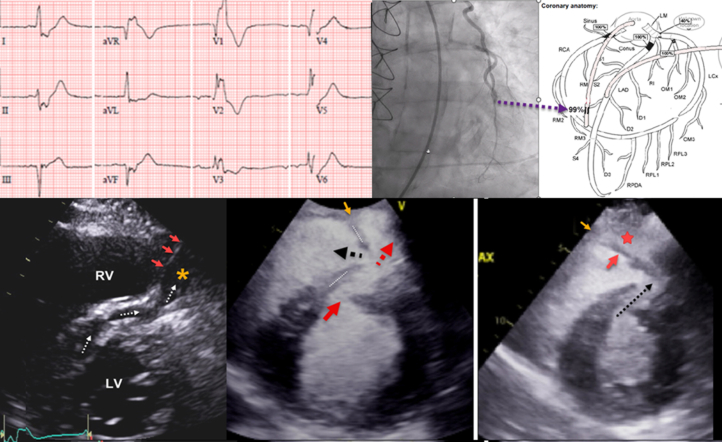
A 77-Year-Old Patient With a History of Coronary Artery Bypass Presented With Myocardial Infarction Two-dimensional echocardiography and echocardiographic contrast-enhanced imaging helped to delineate a ventricular septal defect, with a complex dissection network extending into the right ventricular free wall. LV = left ventricle; RV = right ventricle.
